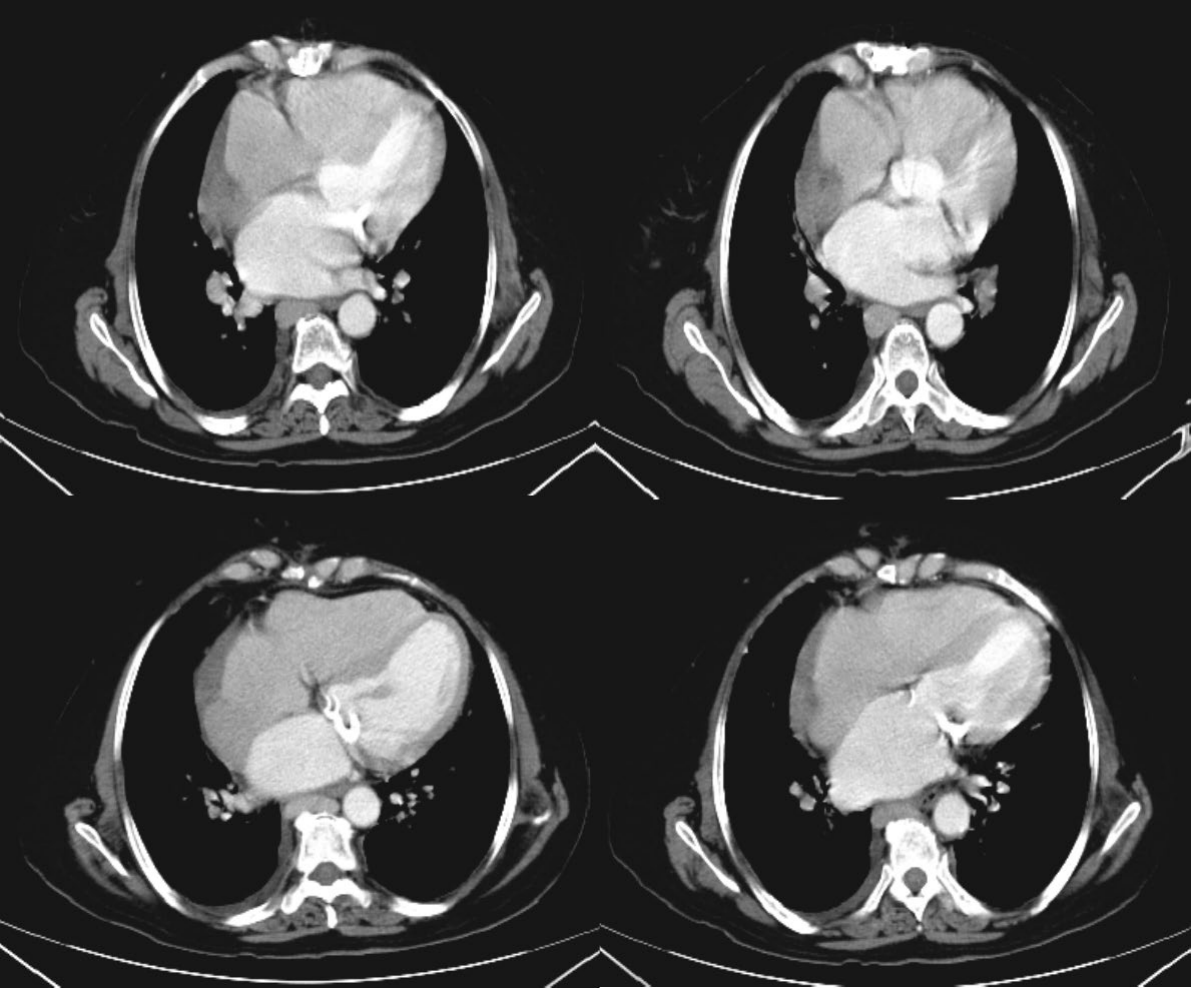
Spiral chest CT scan with contrast: images demonstrate an increase in the size of the mass in the right atrium.
